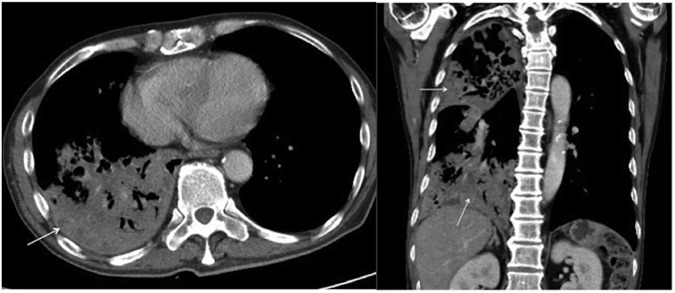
Primary pulmonary tuberculosis pattern with lobar consolidation. A 55-year-old male with culture-proven pulmonary tuberculosis and a history of diabetes mellitus. The patient had a glycosylated hemoglobin (HbA1c) of 9.5%. Axial (A) and coronal (B) contrast-enhanced thoracic CT scans revealed extensive consolidation (arrows) in the right upper and lower lobes.
